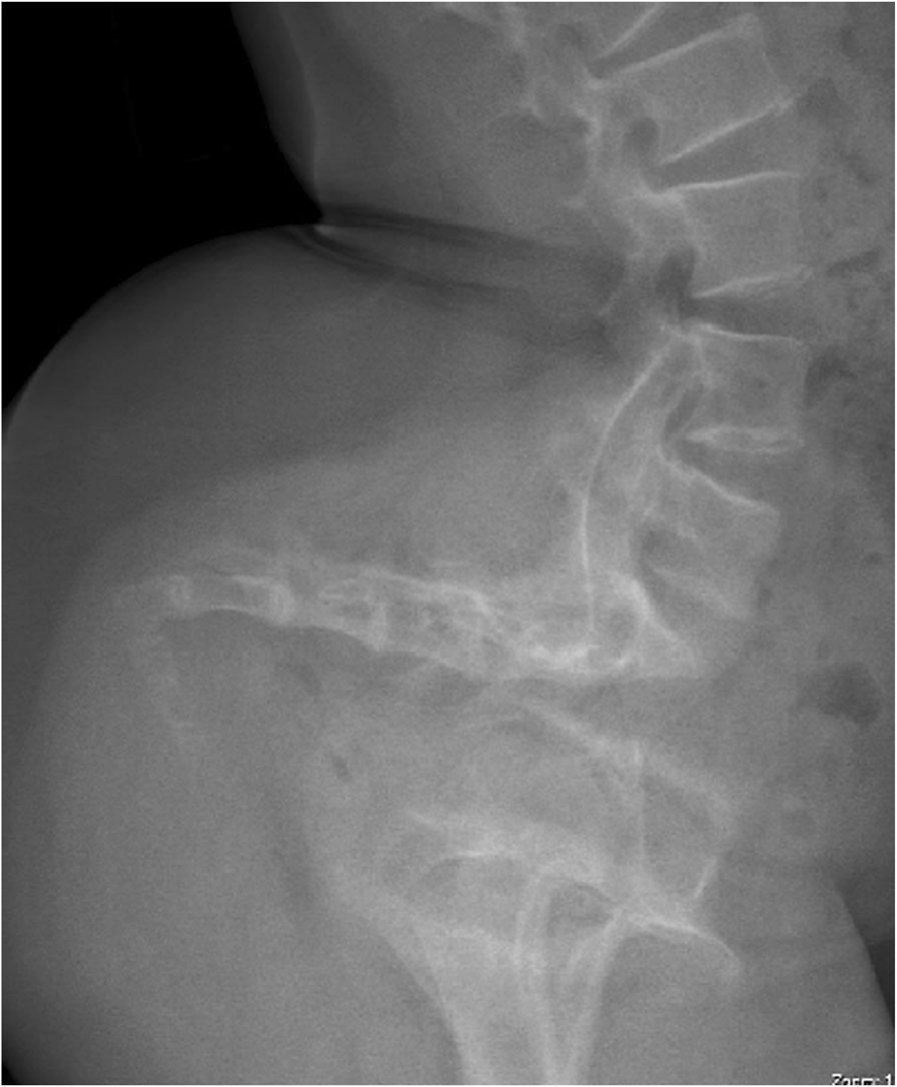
Lateral radiograph of the lumbar and sacral spine. It shows the horizontal sacrum and marked hyperlordosis often seen in those with achondroplasia
